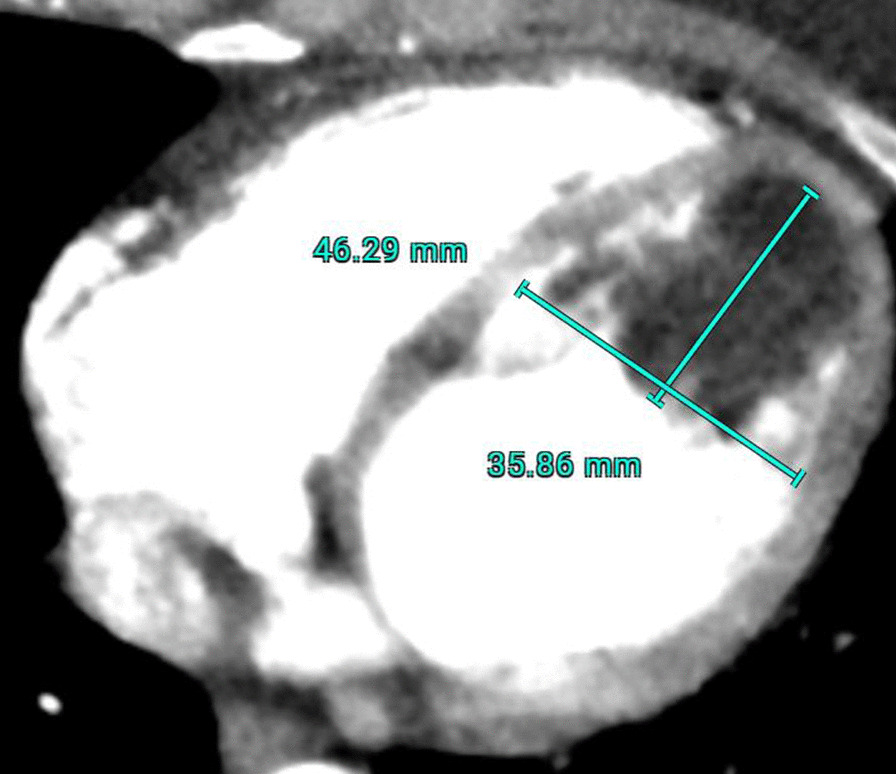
CT image of the LV lipoma
